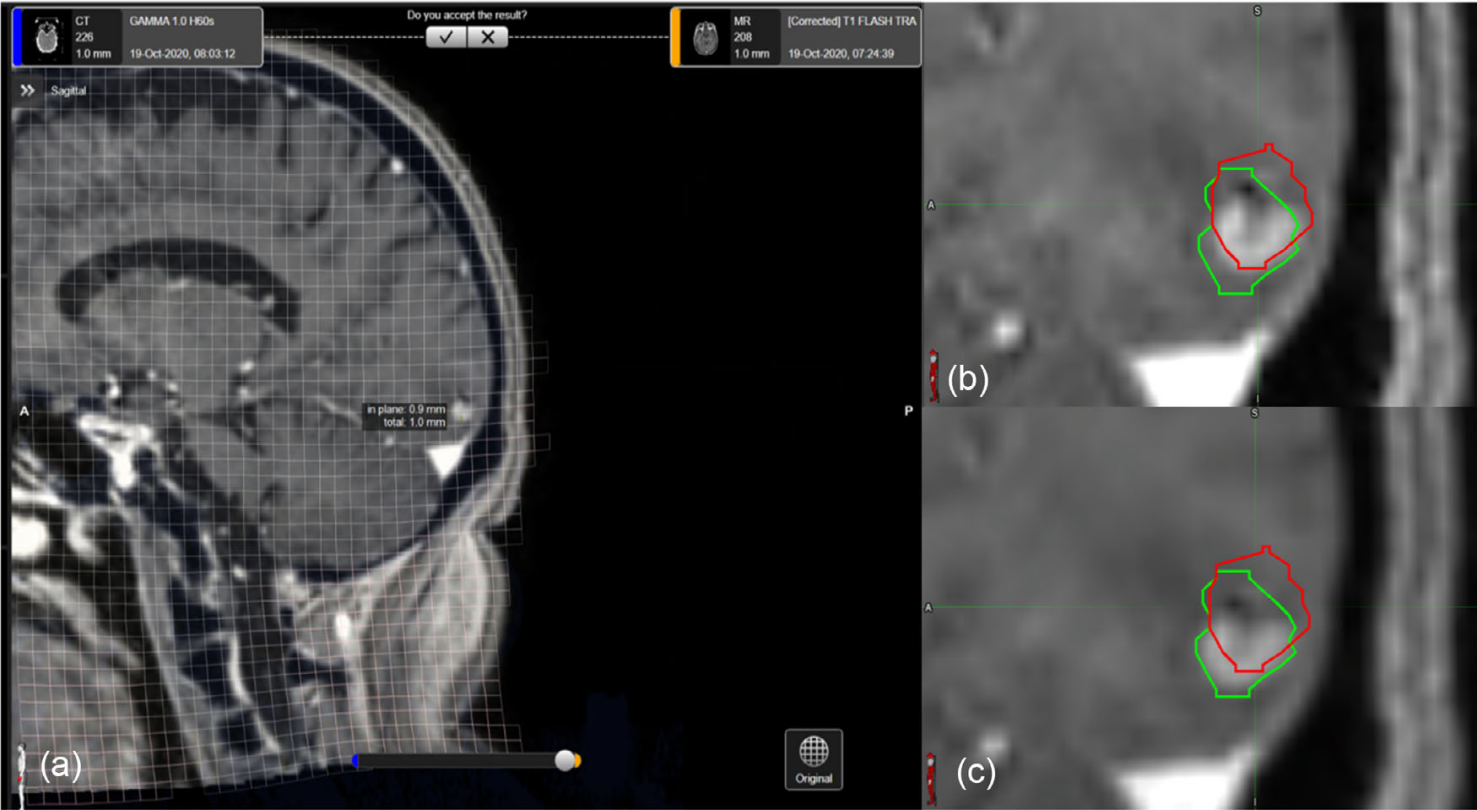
Cranial distortion correction of an MRI using the CDC algorithm. (a) shows the CDC interface displaying the distortion grid. (b) shows the original (red) and corrected (green) contours overlaid on the uncorrected MRI. (c) displays the final corrected MRI.
